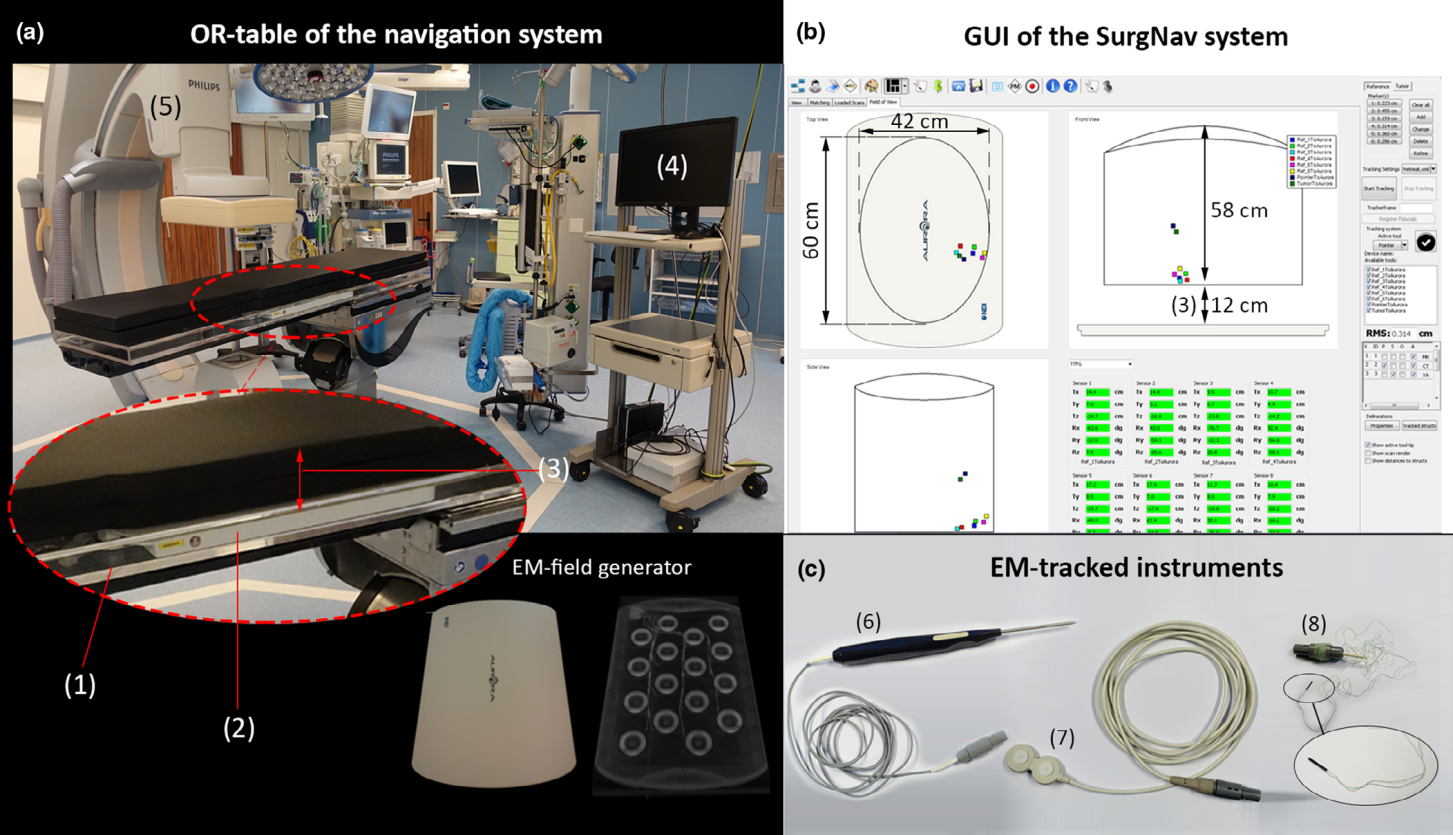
Components of the navigation setup. (a) OR table with a sliding compartment (1) for the EM‐field generator (2), back cushion for elevation of the patient to the level of EM‐tracking FOV (3), navigation trolley (4) and the CBCT scanner (5). (b) Guided user interface of the SurgNav navigation system, visualizing live position of EM‐tracked tools within the FOV of the EM‐field generator. (c) electromagnetically tracked tools of the setup, including EM‐pointer (6), external 5‐DoF patient‐trackers (7) and a micro‐6‐DoF EM‐sensor for tracking of the organ (8).
